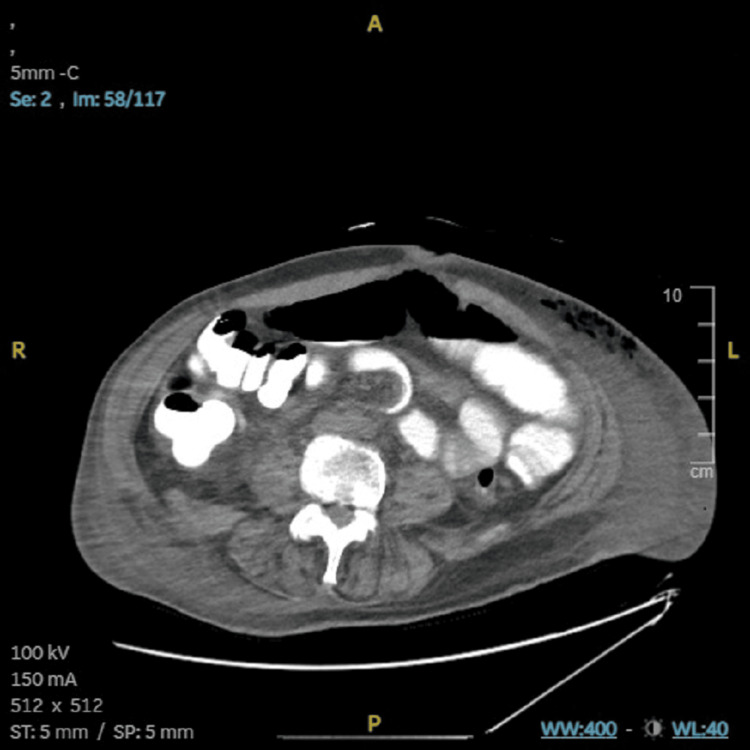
Follow-up contrast-enhanced CT obtained five days after surgical repair, demonstrating free passage of contrast through the bowel to the rectum, with complete resolution of the obstruction and the new gastric tube in a satisfactory position.
